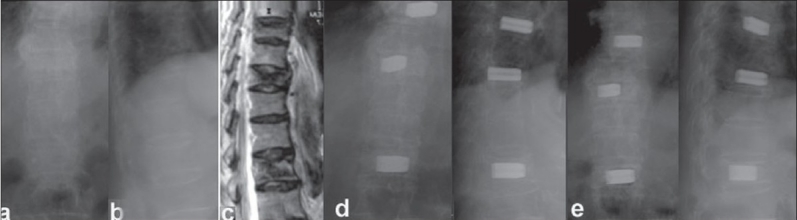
(a-c) Radiograph (anteroposterior and lateral) and T2W sagittal MRI in an 84-years-old female suffered intolerable mid back pain with T8 and T10 costal neuralgia radiating to anterior chest wall showed T8, T10 and L1 osteoporotic compression fractures without cord compression. (d) Radiograph (anteroposterior and lateral view) revealed 3-level TpBA vertebroplasties at initial follow-up. The back pain and costal neuralgia relieved postoperatively. (e) Radiograph (anteroposterior and lateral views) at 30- month follow-up confirmed the good results of TpBA vertebroplasty
